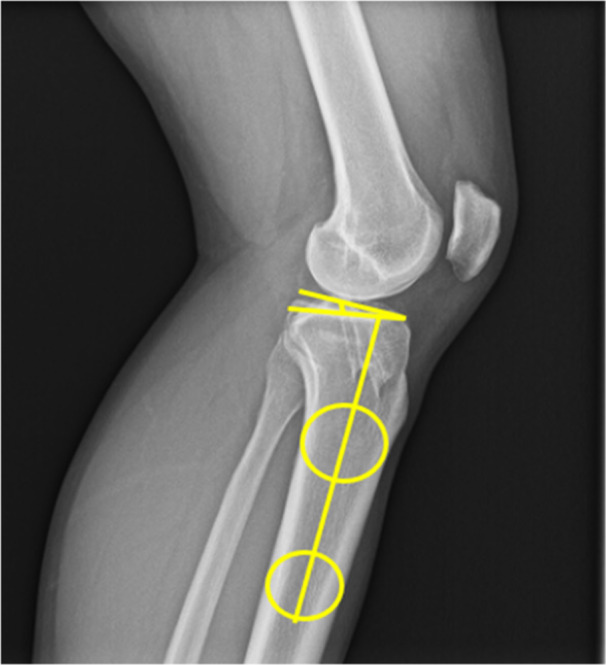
Assessment of the posterior tibial slope (PTS) on a strict lateral radiograph using the centers of two circles placed in the tibial shaft to determine the proximal tibial axis. The PTS was then measured as the angle between an orthogonal line relative to the proximal axis and a tangent of the medial tibial plateau [8].
